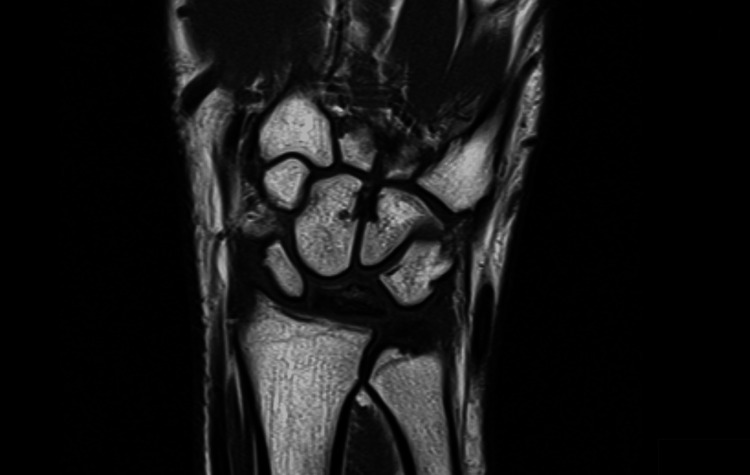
MRI of the left wrist. Coronal T1-weighted MR image shows hypointensity of the lunate bone, confirming the diagnosis of Kienböck's disease.
